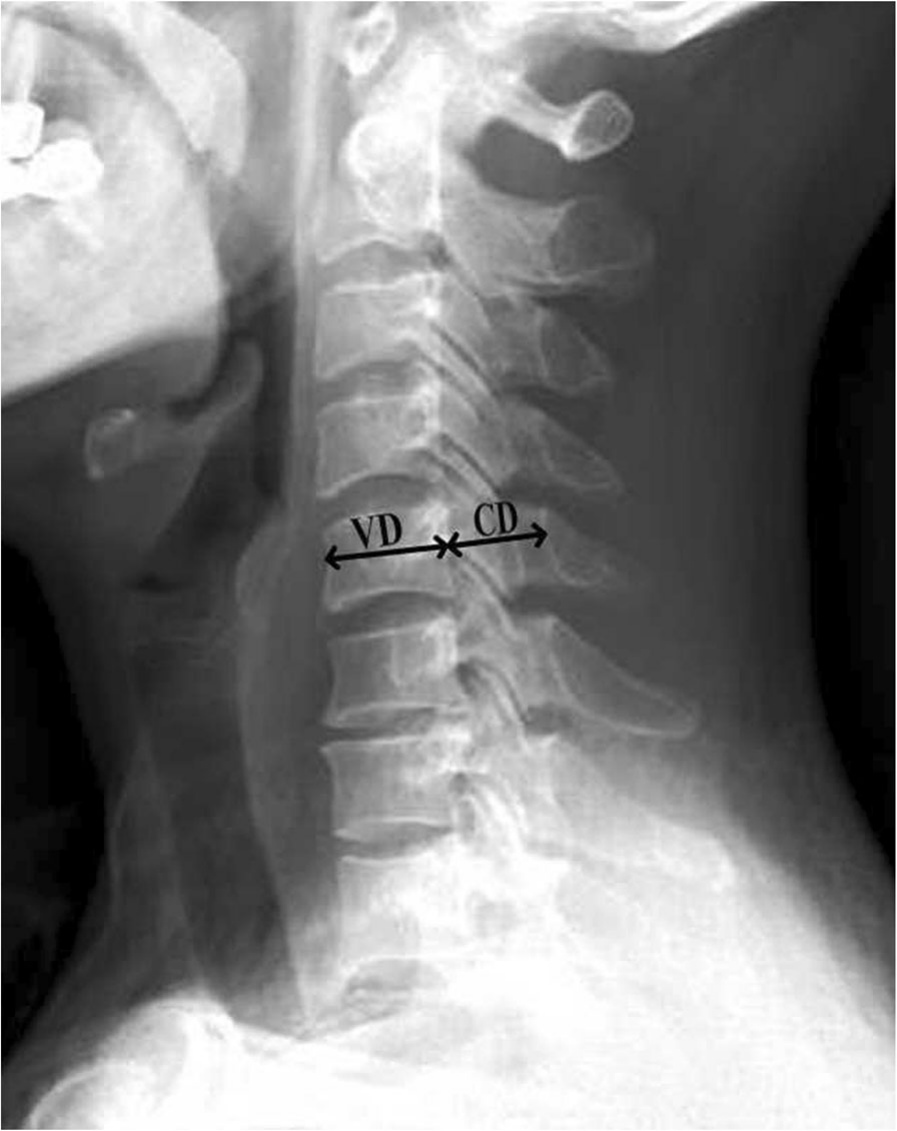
Measurement of the Pavlov ratio on lateral radiography. VD indicated the vertebral diameters, namely, the cervical sagittal diameter of the vertebral body at the midpoint; CD, the canal diameter, namely, the sagittal diameter of the spinal canal from the posterior surface of the vertebral body to the nearest point of the corresponding spinal laminar line. The Pavlov ratio was determined with the formula CD/VD in every level [18]
